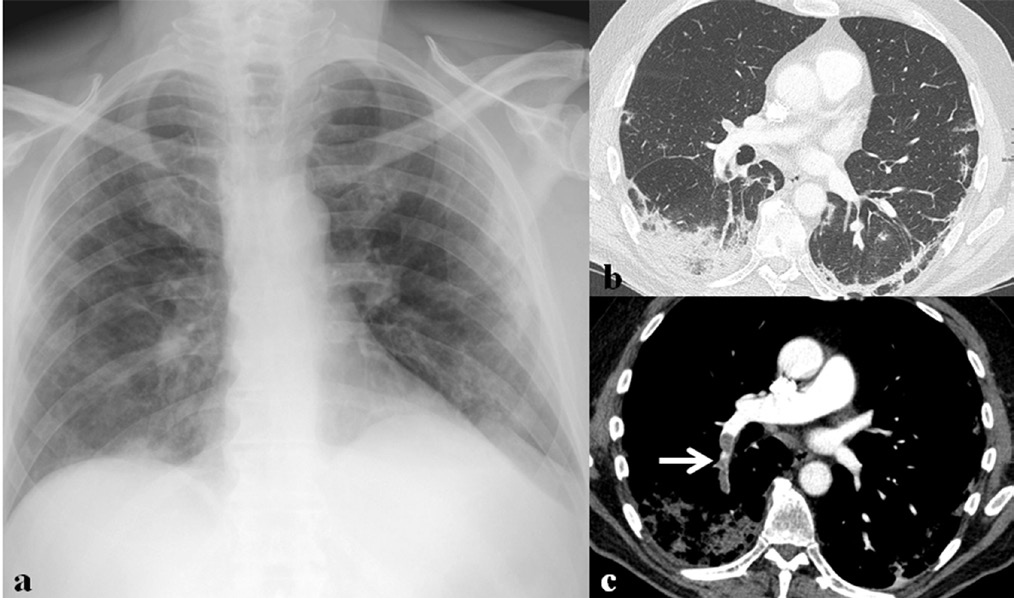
A 71-year-old male patient with SARS-CoV-2 infection. (a) Initial chest radiograph shows bilateral peripheral ground-glass opacities (GGOs) and multifocal patchy consolidation in both lungs. (b) Lung window image of contrast-enhanced chest CT scan obtained on the same day with (a) shows consolidation and patchy GGOs in the subpleural areas of both lower lobes. (c) Mediastinal window image of contrast-enhanced chest CT scan obtained on the same day with (a) shows thromboembolism (arrow) involving right lower lobar pulmonary artery.
